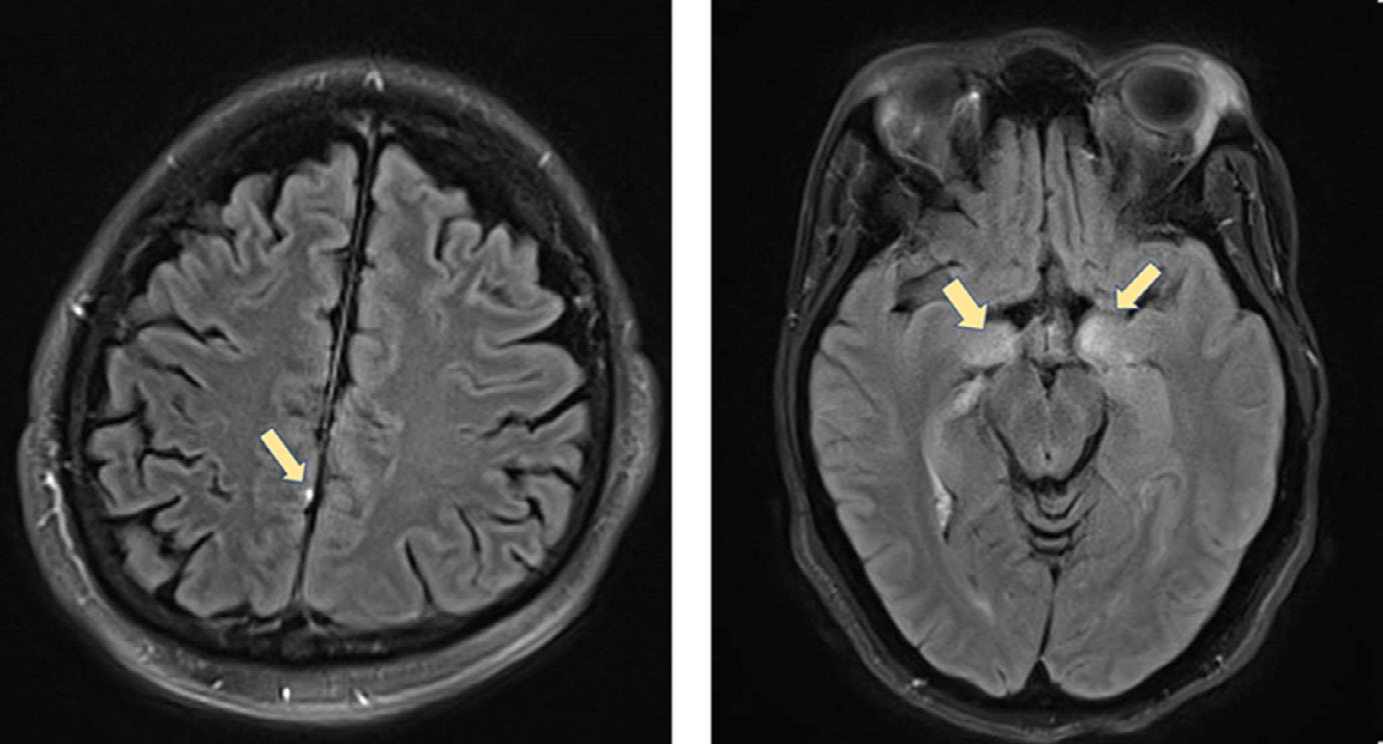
Imaging of patients 1 and 2. (Left) Meningitis, punctate focus of leptomeningeal enhancement of MRI‐Patient 1. Punctate focus of leptomeningeal enhancement on postcontrast FLAIR. (Right) Encephalitis signal abnormality in bilateral mesial temporal lobes on MRI‐Patient 2. Bilateral T2‐FLAIR hyperintense signal in the mesial temporal lobes bilaterally, with associated contrast enhancement
